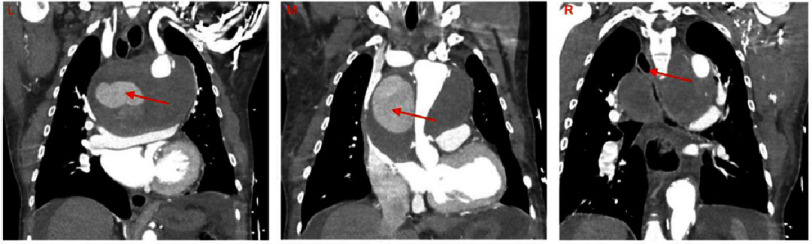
Coronal Images of CT scan of a patient on VV ECMO with an aortic pseudoaneurysm at the distal suture line of a prior aortic arch replacement. Left: Large pseudoaneurysm (arrow) with graft anastomosis visible just distal to the left subclavian, left atrium and heart compressed inferiorly. Middle: Large pseudoaneurysm (arrow) leading to compression of the aortic graft. Right: Large pseudoaneurysm compressing the trachea (arrow) with rightward displacement. Abbreviations: CT - computed tomography, CTA - computed tomography angiogram, VV ECMO - veno-venous extracorporeal membrane oxygenation.
